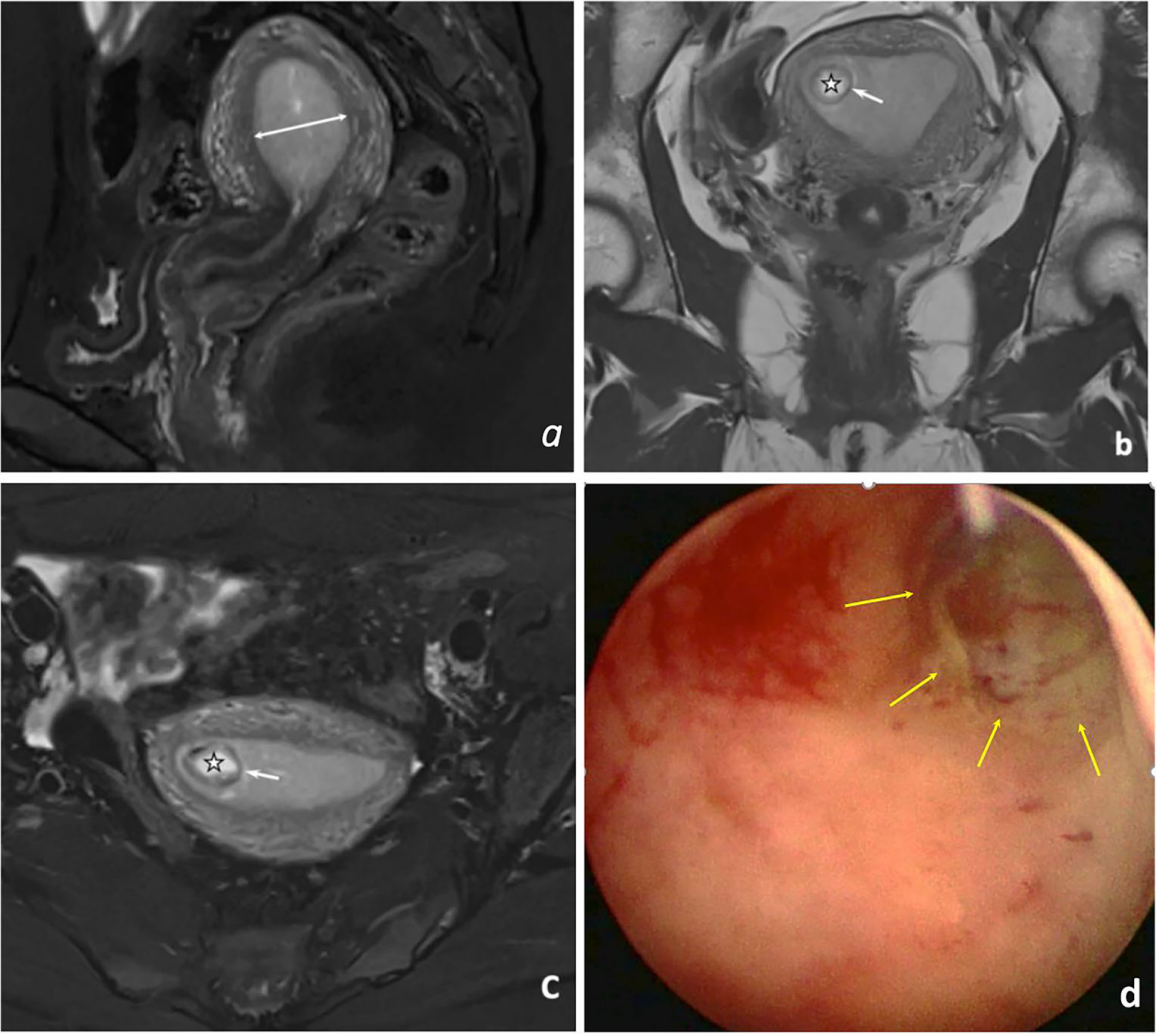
MRI and hysteroscopy in a 24-year-old patient with upper-lateral intracavitary pregnancy. a Sagittal fat-suppressed T2WI showing an endometrial thickness (double-ended arrow) of 22.0 mm. b Coronal T2WI and (c) axial fat-suppressed T2WI showing the gestational sac (star) and medial free edge (arrow). d Hysteroscopic view showing the intrauterine exposed portion of the gestational sac forming a free edge (yellow arrows)
